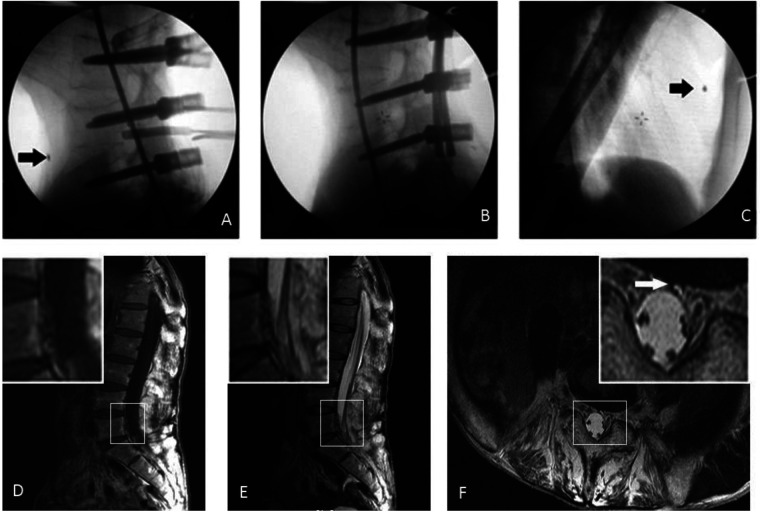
Intraoperative x-ray revealed that the broken metal tip (arrow) was located in the right anterior between the fourth and fifth lumbar vertebrae (A). After L2 vertebral body osteotomy, the metal fragment was found to migrate to the heart (B,C). MR imaging showed abnormal internal vertebral venous plexus in the dorsal part of the 5th lumbar vertebral body, on T1W (D), T2W (E), and axial (F) images. MR, magnetic resonance.
